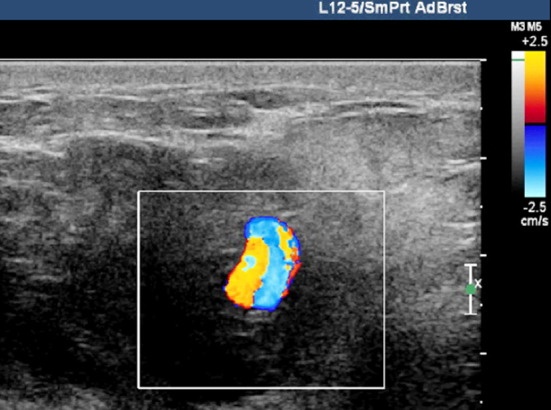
Color Doppler ultrasound of right breast pseudoaneurysm combined with hematoma.
